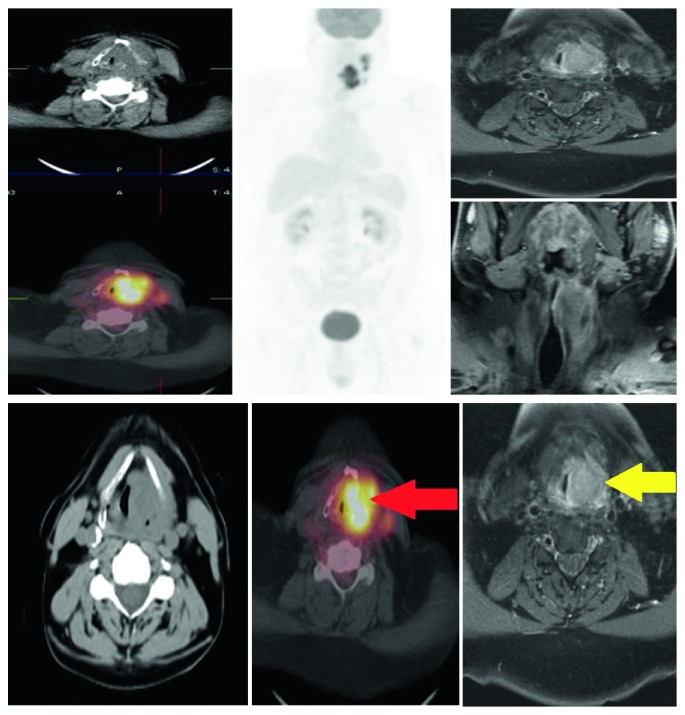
Pt T4 (cartilage infiltration): PET/CT positive (TP, red arrow) vs. MR positive (TP, yellow arrow). SUVmax, 18.56; MTV, 43.03 cm^3^.
